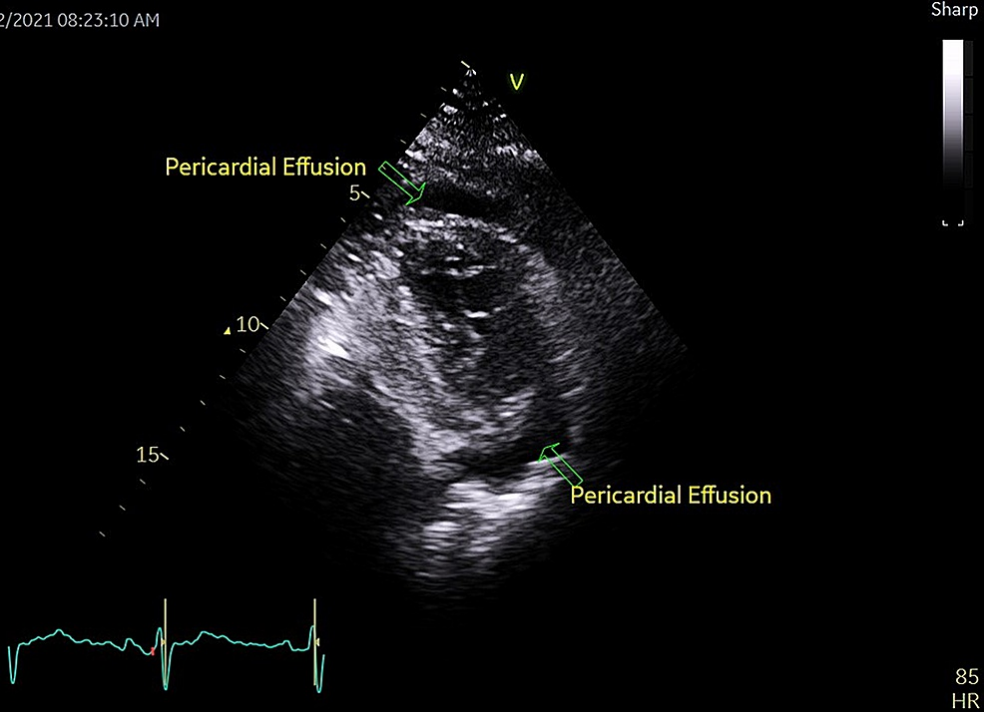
Transthoracic echocardiography images (February 2021)
